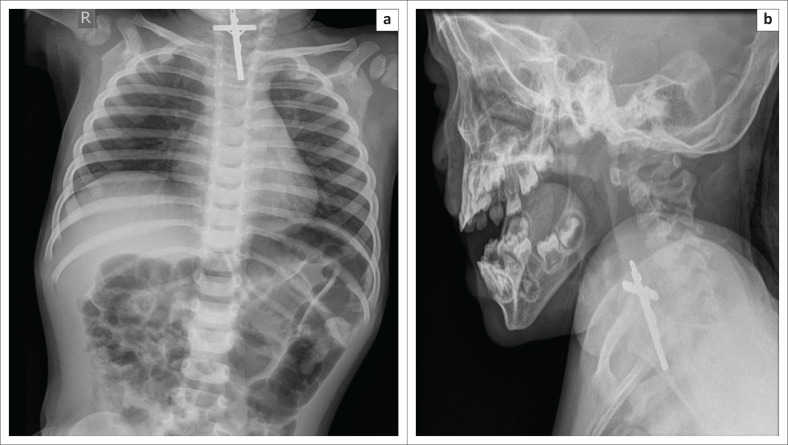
Frontal (a) and lateral (b) radiographs of the neck indicates a crucifix impacted in the cervical oesophagus of a 2-year-old child. The foreign body was retrieved by rigid oesophagoscopy.
